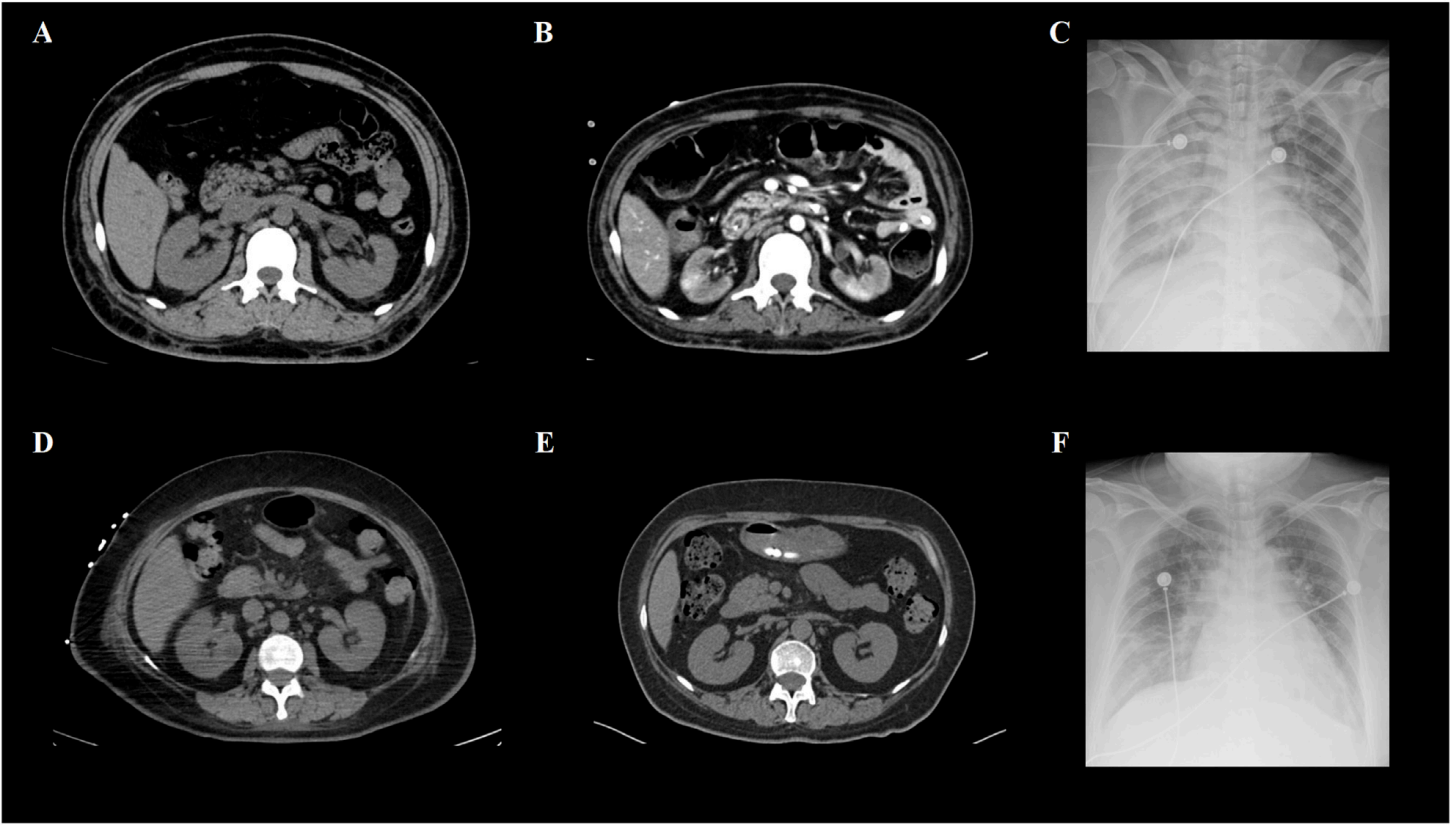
CT and X-ray images of two APL patients with renal failure. (A) Abdominal CT of patient #2 revealed kidney swelling and enlargement when DS occurred. (B) Patient #2 had persistent renal dysfunction and became dialysis-dependent after achieving complete remission of APL. Enhanced CT imaging 3 months later revealed kidney shrinkage and decreased contrast intake at focal sites, which was consistent with irreversible changes in the renal function. (C) Obvious bilateral pulmonary infiltrates caused by DS were demonstrated by the chest X-ray of patient #2. (D) Abdominal CT of patient #5 also revealed kidney swelling and enlargement in the DS. (E) Unlike patient #2, the renal function of patient #5 was restored to normal after DS was successfully managed. Non-contrast CT imaging 2 months later revealed that both kidneys had normal appearances. (F) Similar to patient #2, patient #5 developed pulmonary infiltration, shown as patch opacities on the chest X-ray at the pinnacle of the DS.
